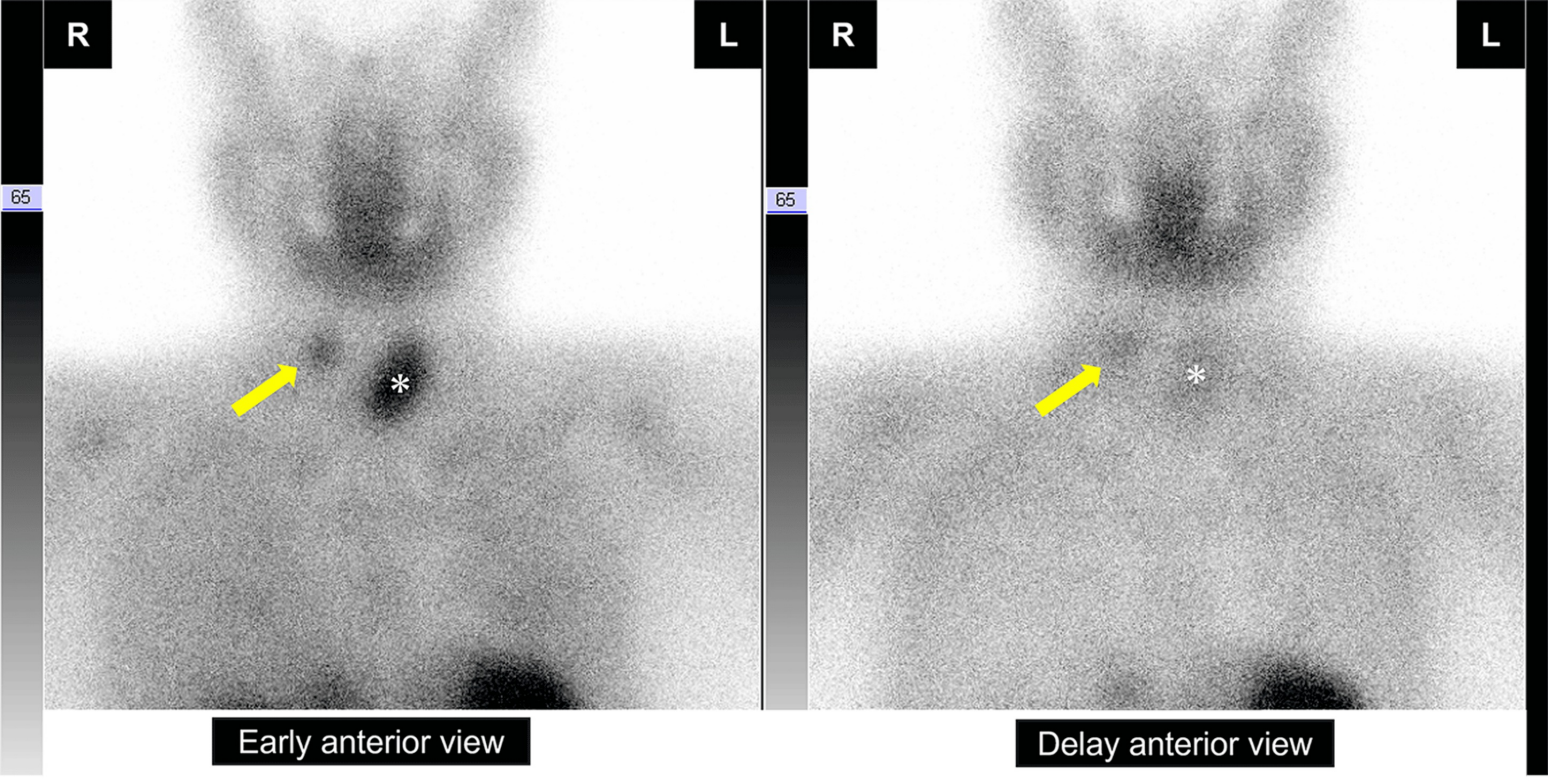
Technetium-99m sestamibi scintigraphy Technetium-99m sestamibi scintigraphy shows increased uptake in the right parathyroid gland (arrow). The asterisk indicates the left lobe of the thyroid gland.
